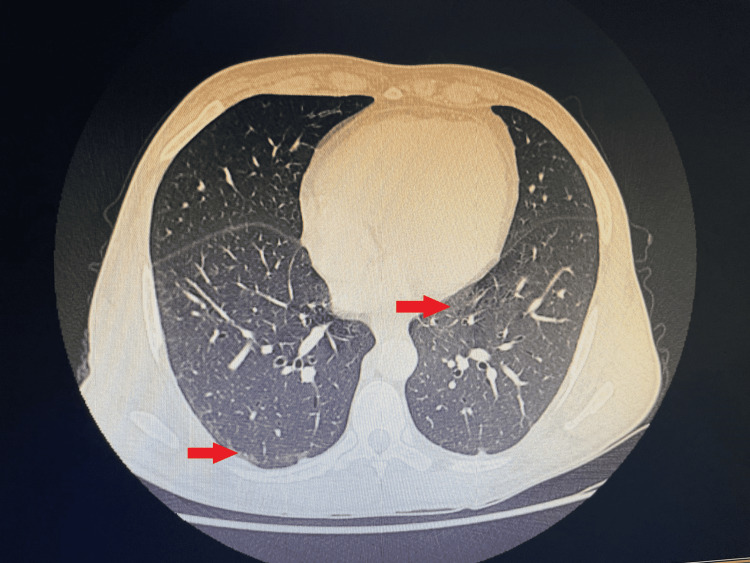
Thoracic computed tomography showing bilateral interstitial (red arrows) lung disease in cross-section
